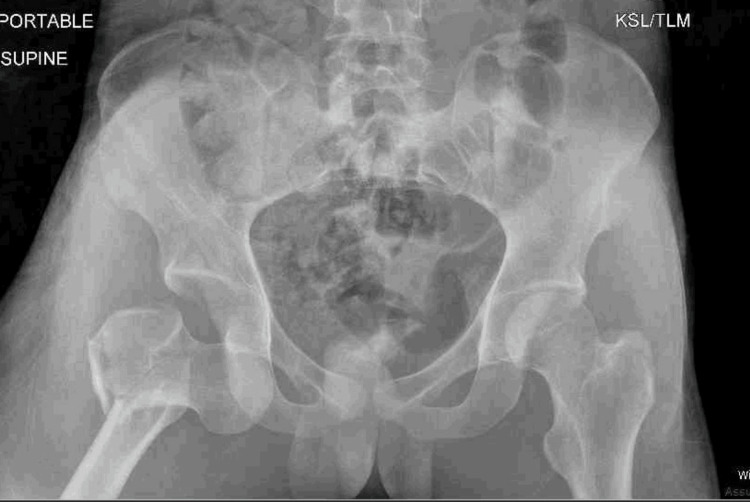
Anteroposterior (AP) radiograph showing direct inferior hip dislocation with an associated intertrochanteric fracture The obturator ring remains unobstructed in contrast with an anterior-obturator dislocation.
